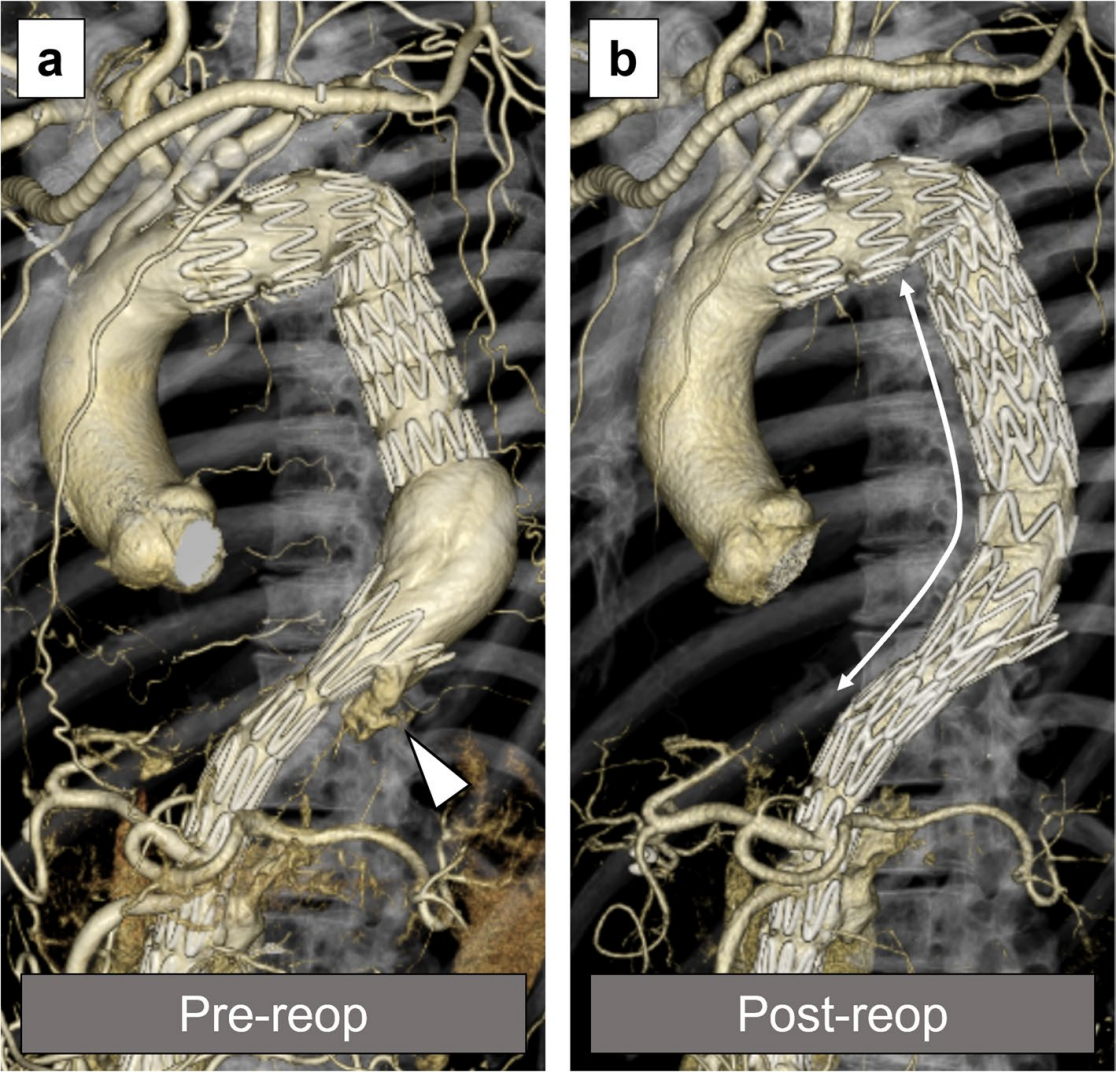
Pre- and postoperative computed tomography (CT) images of the second TEVAR. a Descending aortic aneurysm with stent-induced new entry (SINE) and device migration; 9 years had passed since the onset of the aortic dissection. The white arrowhead indicates the SINE. b postoperative CT scan after the second TEVAR showing that the SINE disappeared. The white line represents the length of the new stent-graft
